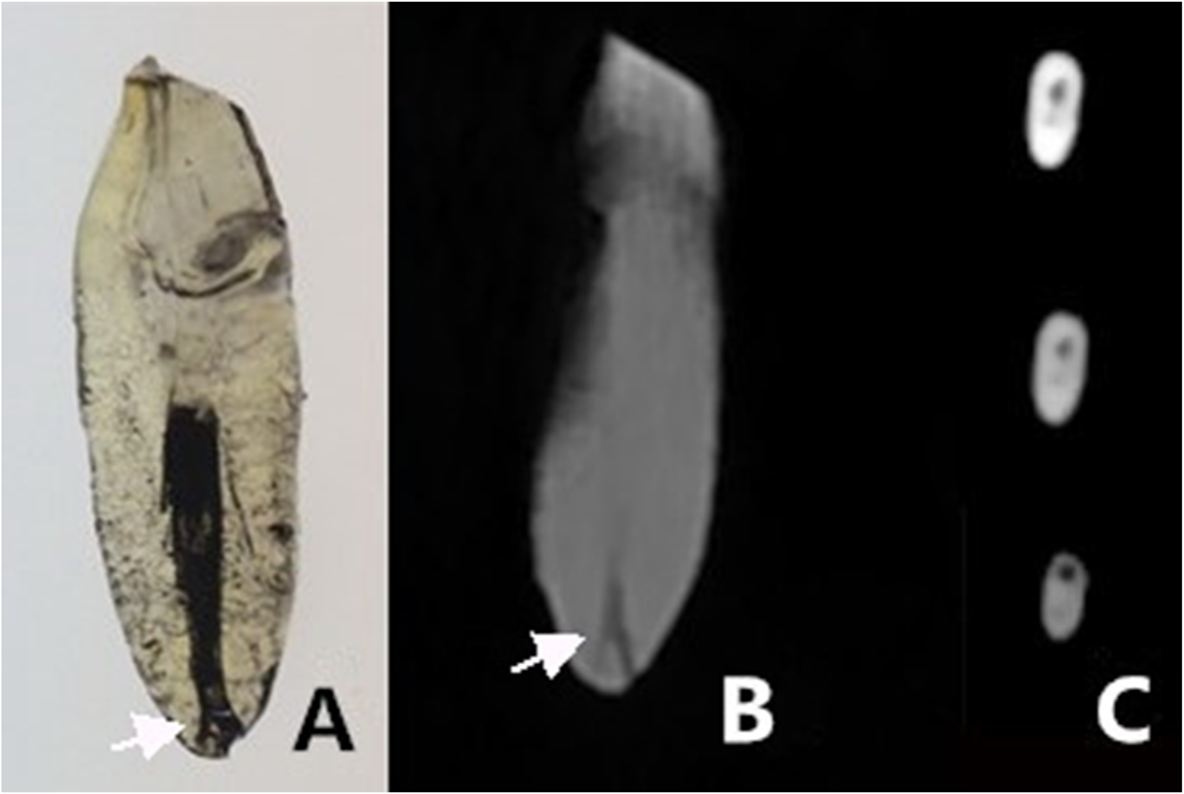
Image of a tooth with apical deltas. a Cleared tooth demonstrating the apical delta. b CBCT image with MPR reconstruction. c CBCT images in the axial view. The main root canal can be seen migrating to the lingual side of the root. A subtle branch can be seen extending from the main root canal to the labial side of the root
